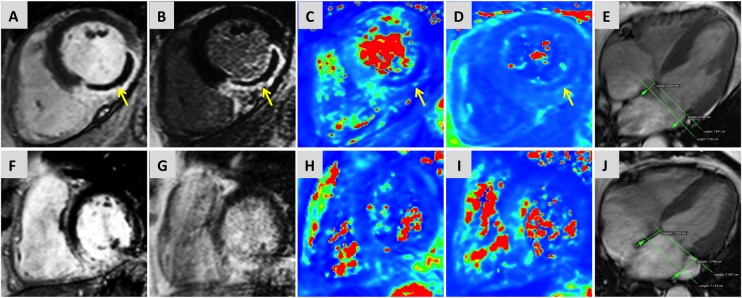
Multiparametric CMR examination of two cases of ST-elevation myocardial infarction. Case 1 (top row): Case of inferior-lateral MI with presence of MVO on early gadolinium enhancement imaging (A) and LGE (B). IMH within MVO was confirmed on T2-weighted maps (C) and T2*-maps (D). 4-chamber cine imaging (E) confirmed reduced averaged-MAPSE (overlay of systolic and diastolic annular position shown in green). Case 2: Case of inferior MI without the presence of MVO (F and G) or IMH (H and I) and comparatively better longitudinal displacement than case 1 (J). CMR, cardiovascular MR; IMH, intramyocardial haemorrhage; LGE, late gadolinium enhancement; MAPSE, mitral annular plane systolic excursion; MVO, microvascular obstruction.
